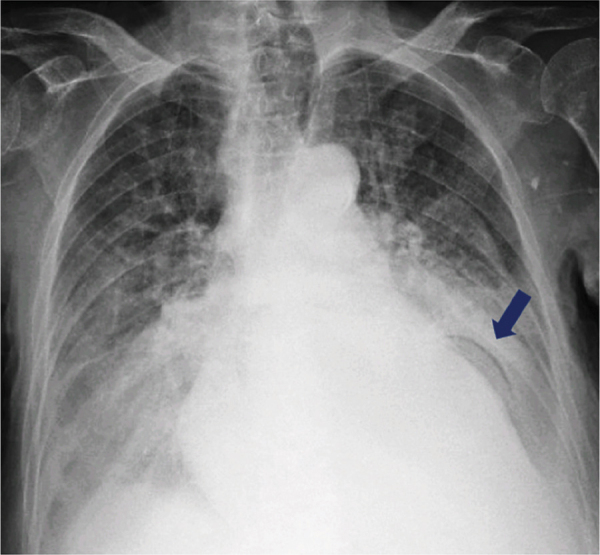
Follow-up chest radiograph showing gastric pneumatosis as a linear lucency outlining the circumference of the intrathoracic stomach (arrow).
